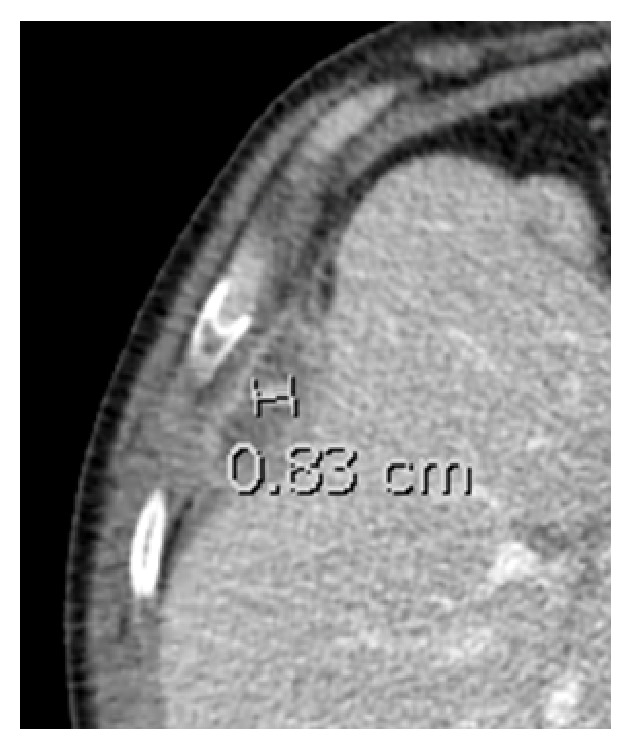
Retained gallstone 5 years following laparoscopic cholecystectomy visualized with computed tomography with a 1 cm radiopaque density within perihepatic fluid collection.
